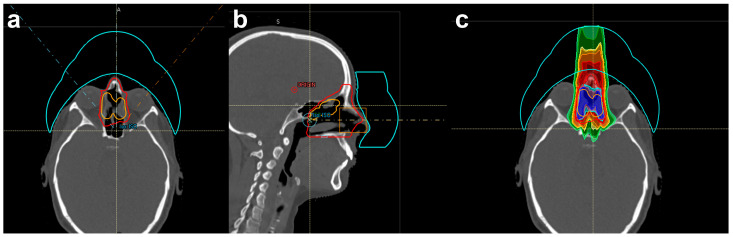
Beam configuration and dose distribution with patient-specific bolus for cancer patients treated with proton therapy. (a,b) Axial and sagittal CT views of a two-field arrangement for central tumour localisation, using a custom bolus. (c) Corresponding dose distribution.
